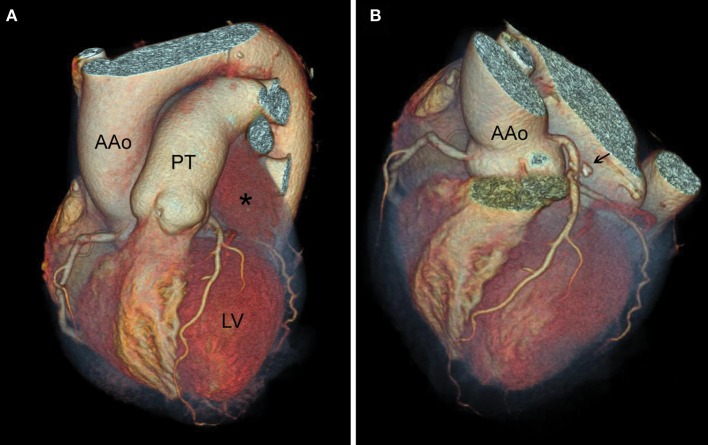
Heart computed tomography, 3-dimensional reconstruction. (A) The tumor (asterisk) is located posteriorly and laterally to the pulmonary trunk (PT) and the ascending aorta (AAo), superiorly to the left ventricle (LV). (B) Macrocalcificacion (black arrow) as a part of the tumor's structure.
